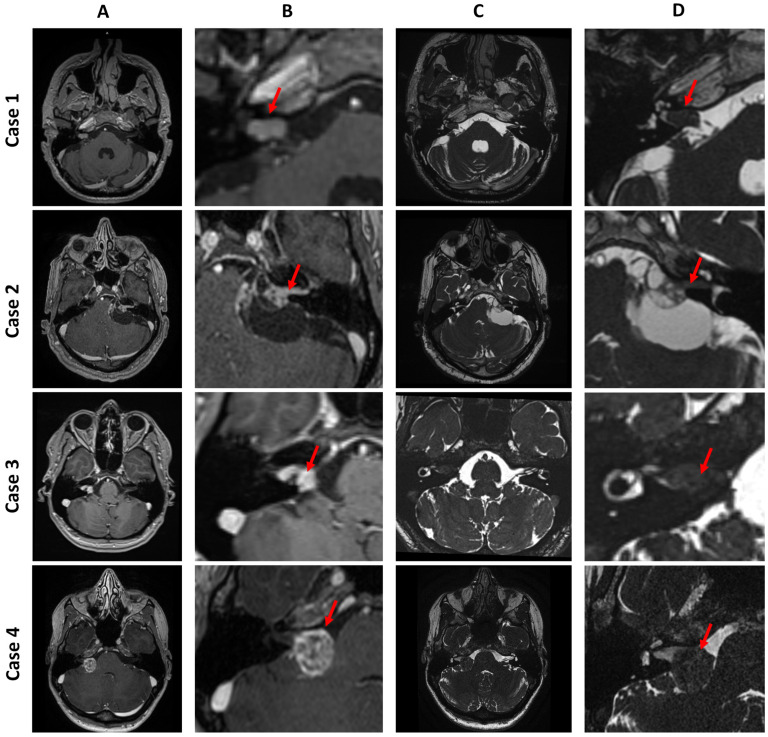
Four cases of vestibular schwannoma presented in four consecutive rows. Column (A) and column (B) show a general and detailed view of T1-weighted images with contrast enhancement. Column (C) and column (D) show a general and detailed view of T2-weighted images. The red arrow points to the tumor.
